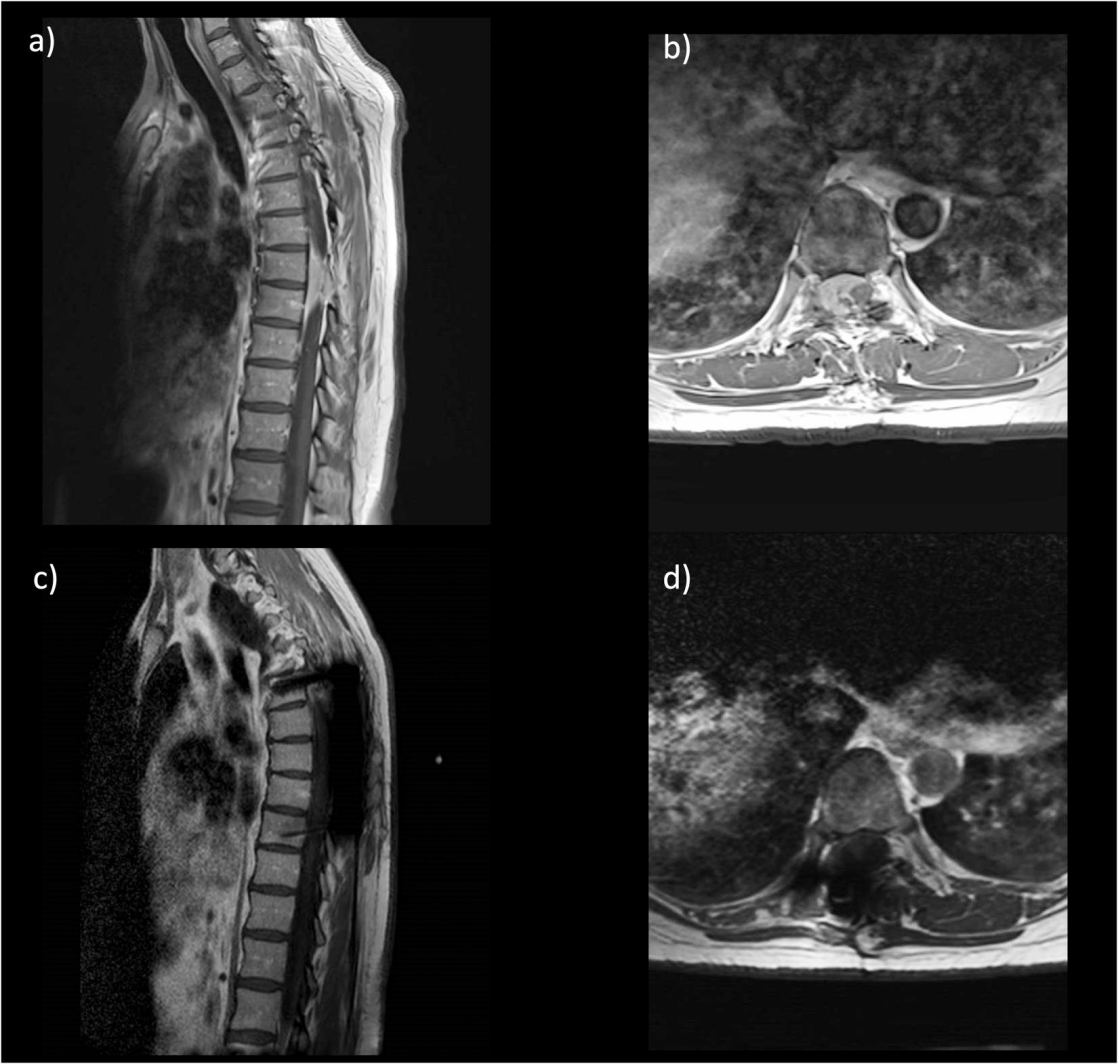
Case of benign thoracic spine tumour with pre-operative contrast MRI images showing the recurrent thoracic meningioma in sagittal (a) and axial (b) views. Post-operative images show satisfactory resection in sagittal (c) and axial (d) views.
